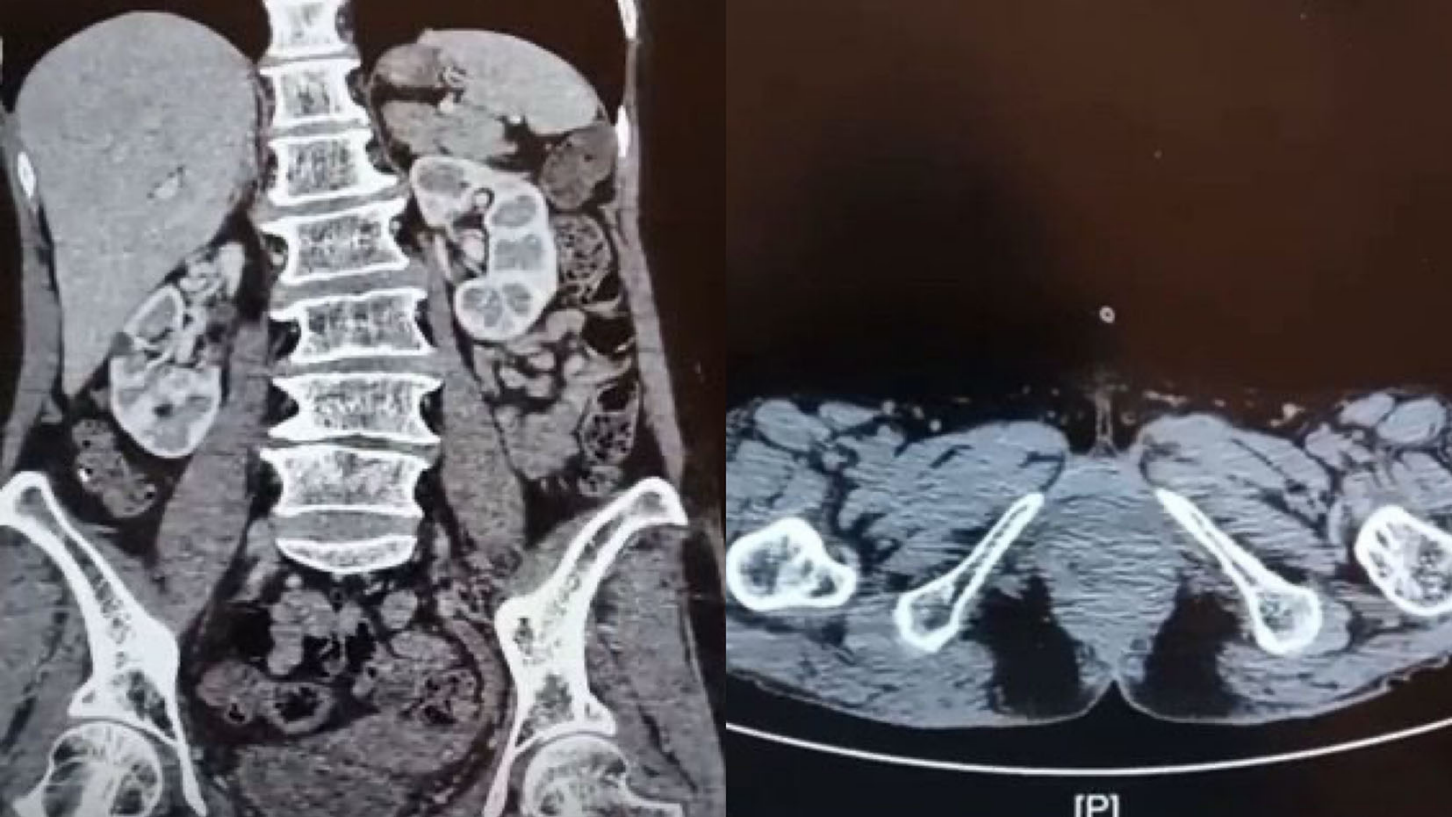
CT scan revealed a suspected malignant mass in the entire length of urethra, with no inguinal lymph nodes enlargement and no evidence of metastases.
